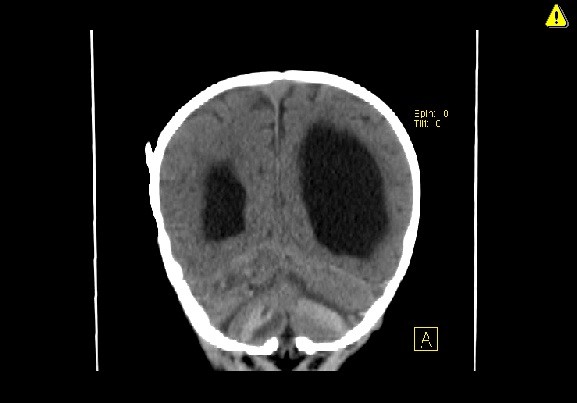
Coronal computed tomography scan demonstrating bilateral cerebellar hemorrhages.
